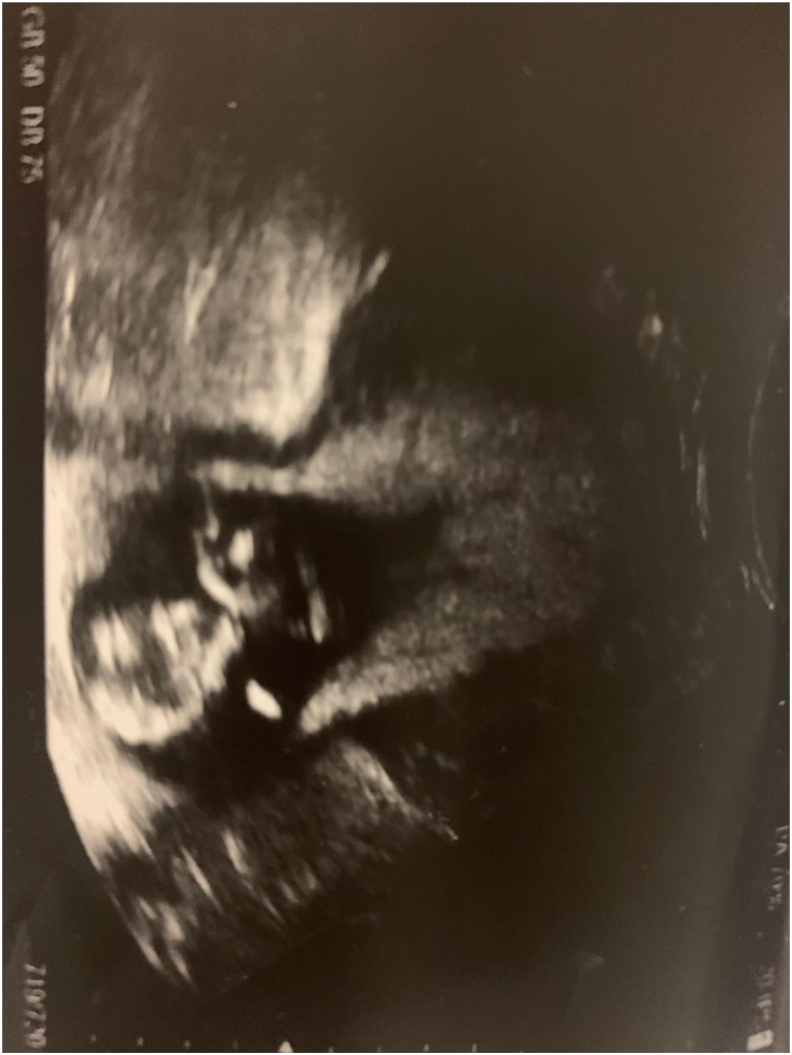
F2 abdominal ultrasound scan showing an evolving mono-fetal pregnancy with an estimated weight of 1 kg and a.
